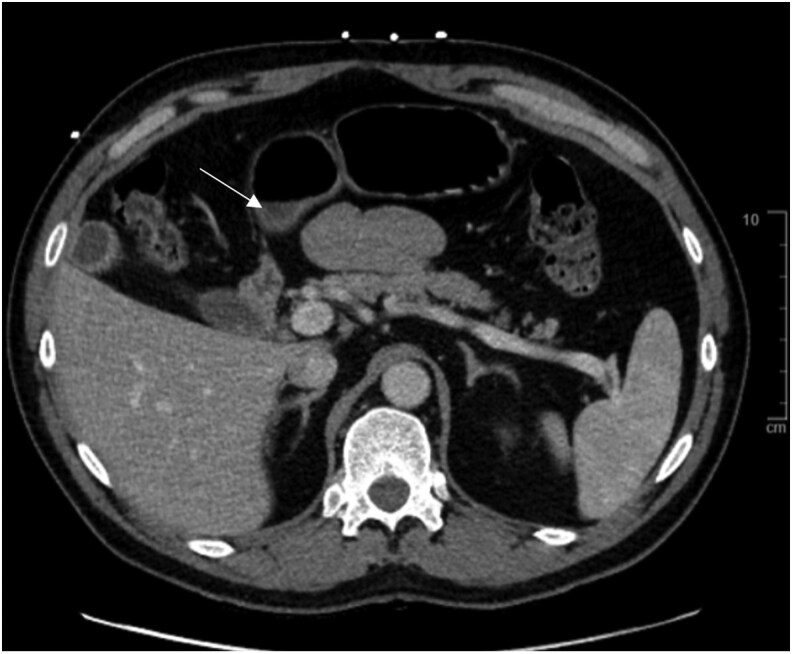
CT imaging assessment for insulinoma or pancreatic mass. Contrast-enhanced CT of the abdomen and pelvis demonstrates postsurgical changes at the gastroesophageal junction and posterior gastric wall (white arrow). The pancreas is normal in appearance, with no masses or focal lesions identified in the head, body, or tail. No findings concerning for insulinoma were visualized. Abbreviation: CT, computed tomography.
